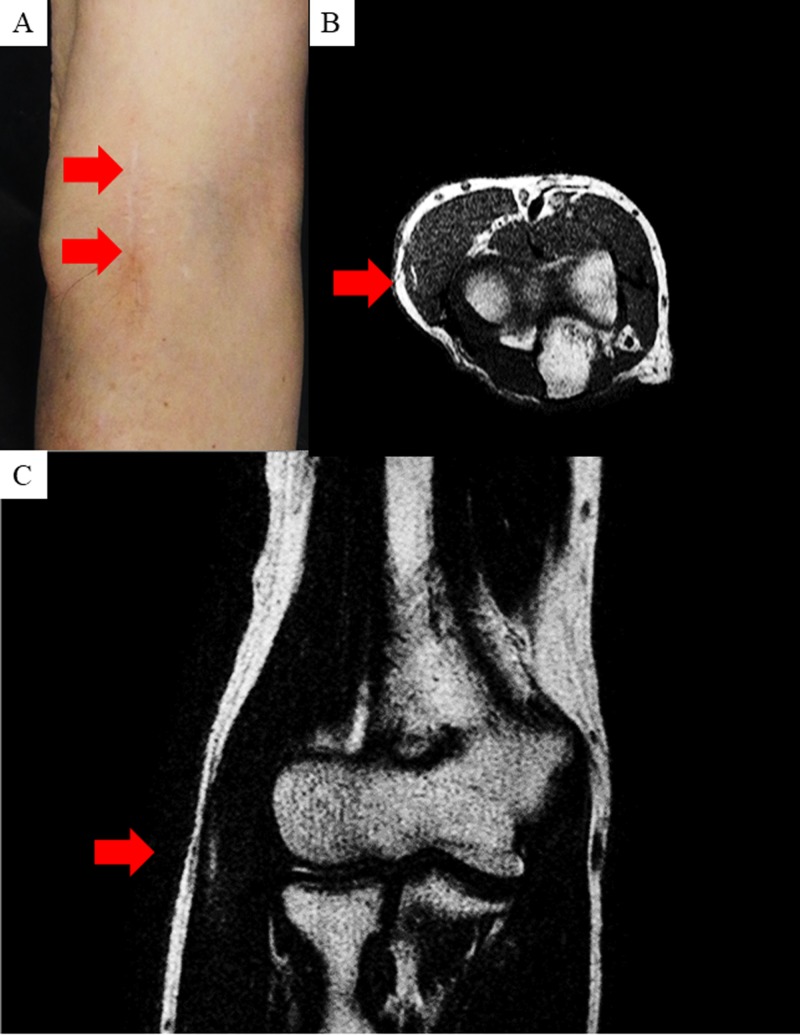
Appearance and magnetic resonance imaging (MRI) features of the right elbow. Appearance of the right elbow after the first resection (A). MRI shows the right elbow after the first resection with coronal (B) and sagittal sections (C). Small region of high intensity is observed and they favor some inflammation after the surgery (B and C).
